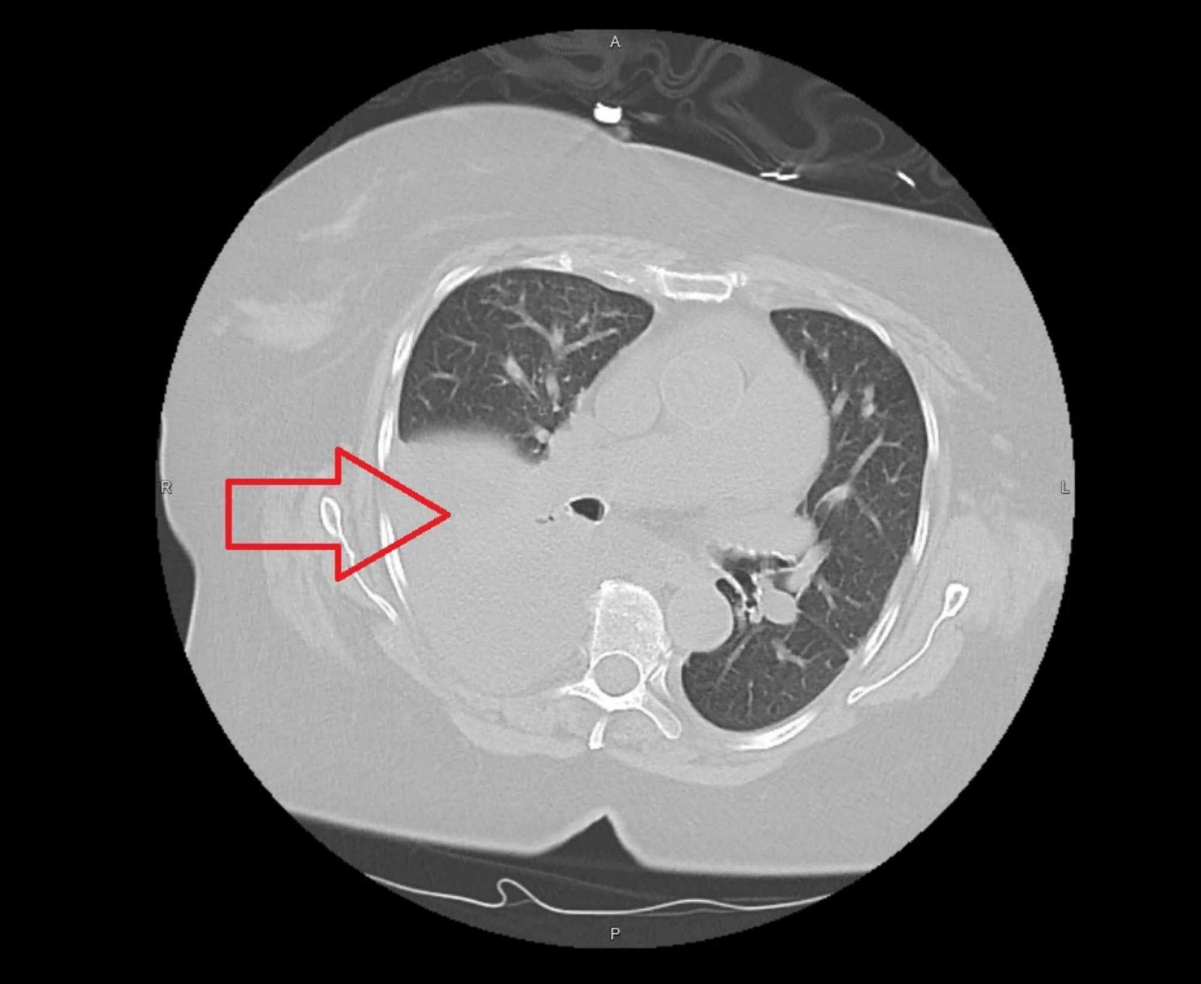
CT chest without contrast showing large right lung effusions (red arrow) with atelectasis and cardiomegaly
